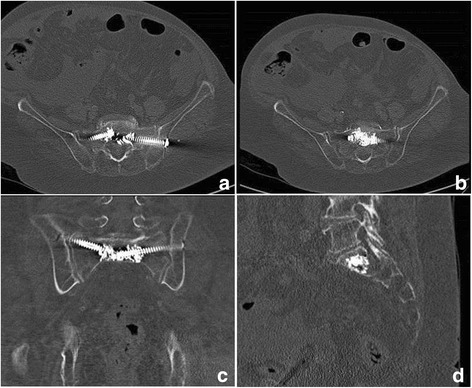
Postoperative pelvic CT of in-screw cement-augmented SI screw fixation in axial (a and b), coronal (c) and sagittal (d) views
